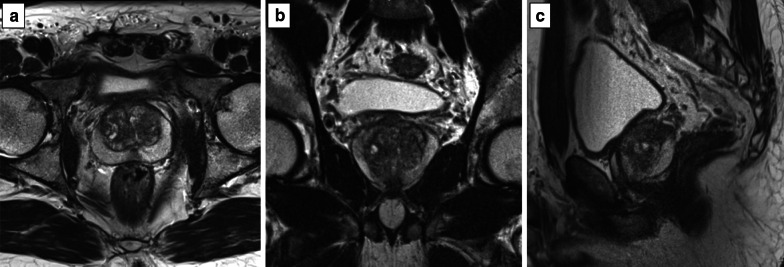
T2-weighted images obtained in the axial (a), coronal (b) and sagittal (c) planes
